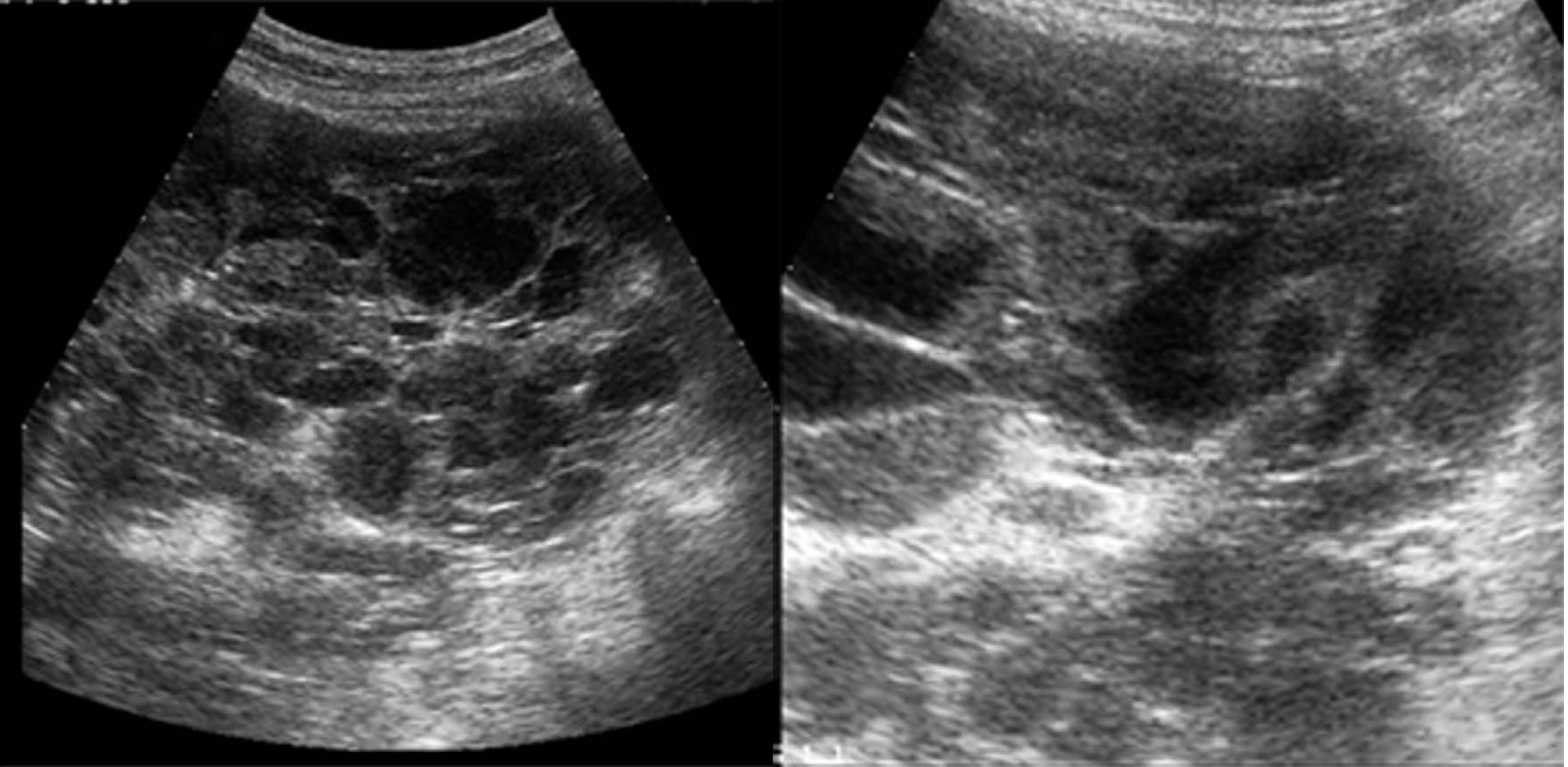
Sagittal gray-scale ultrasound scan of right kidney obtained after repeated irrigation and drainage demonstrates decreasing amount of internal echoes and septa in the cysts.
